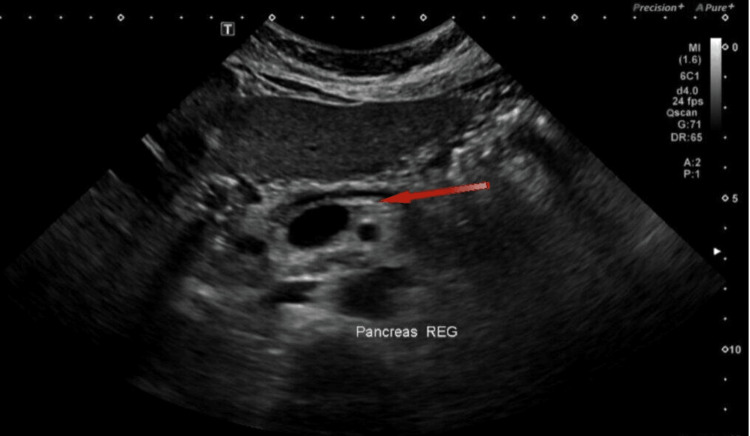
Ultrasound of the abdomen demonstrating normal pancreas echotexture with prominent main pancreatic duct (red arrow)
